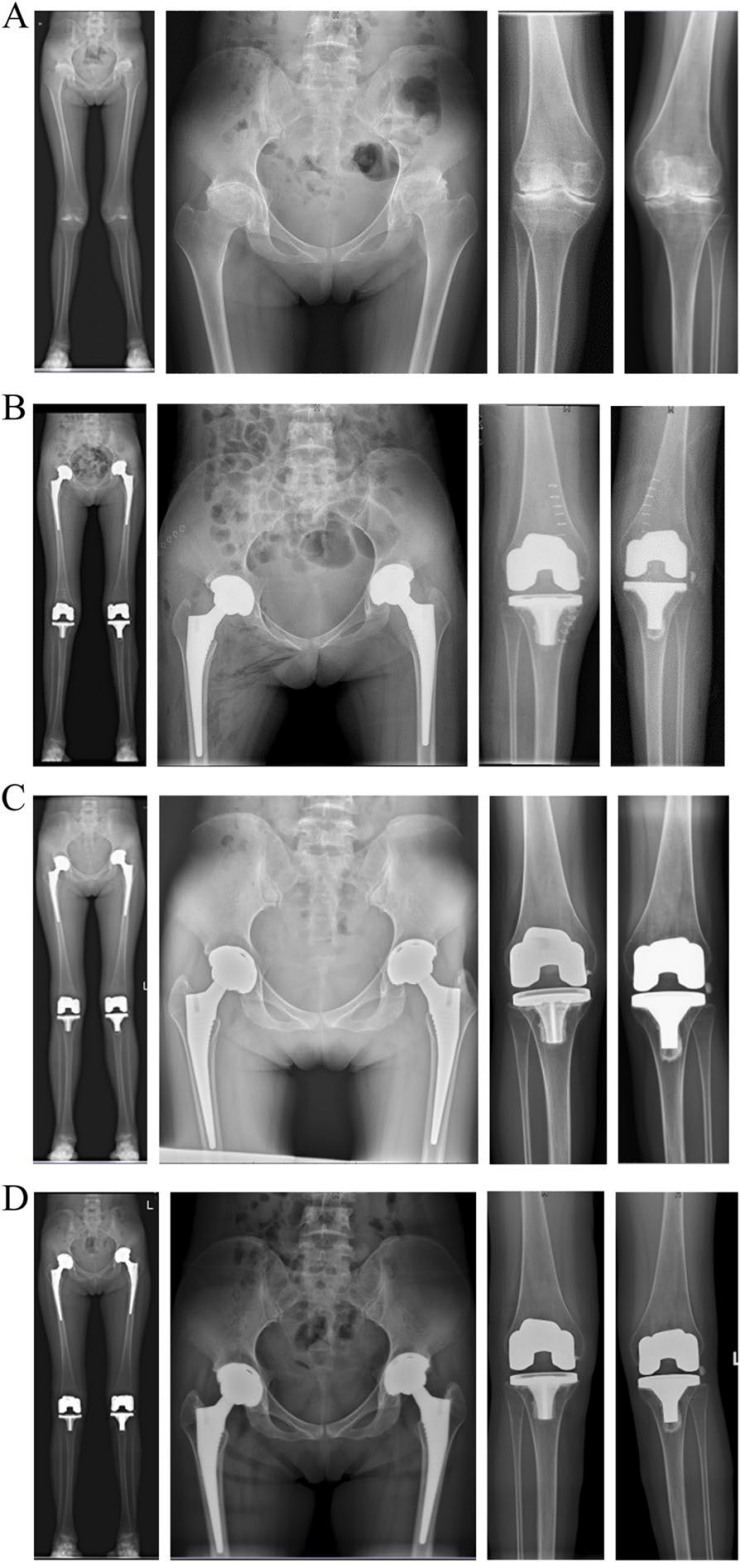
Radiographs of a 26-year-old woman with 16-year rheumatoid arthritis who underwent bilateral cementless total hip arthroplasty and cemented posterior-stabilized total knee arthroplasty. The operation sequence was left knee, left hip, right hip, and right knee, with the surgeries separated by five, eight, and two months. A Preoperative radiographs show uniform joint space narrowing in the bilateral hips and knees. B Early postoperative radiographs. C, D Postoperative radiographs were taken at the mean of 62 and 108 months, showing all components to be stable
